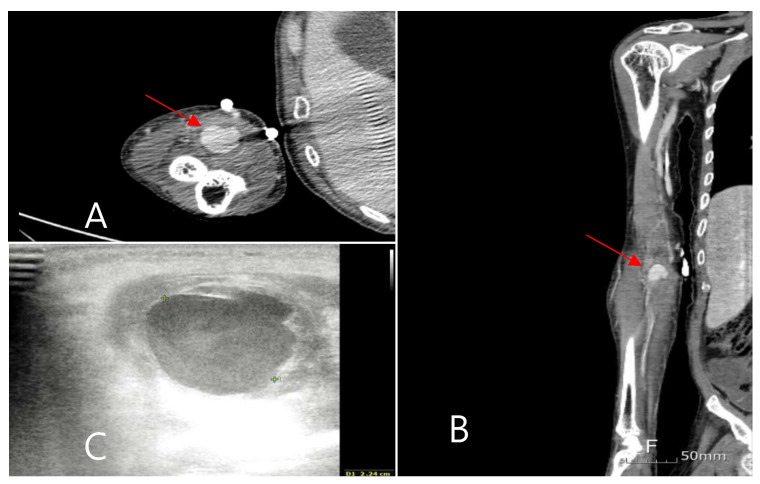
(A,B): Brachial artery mycotic aneurysm is observed in upper extremity CT. (C): Thrombosis and cellulitis around aneurysm are examined with Doppler.
